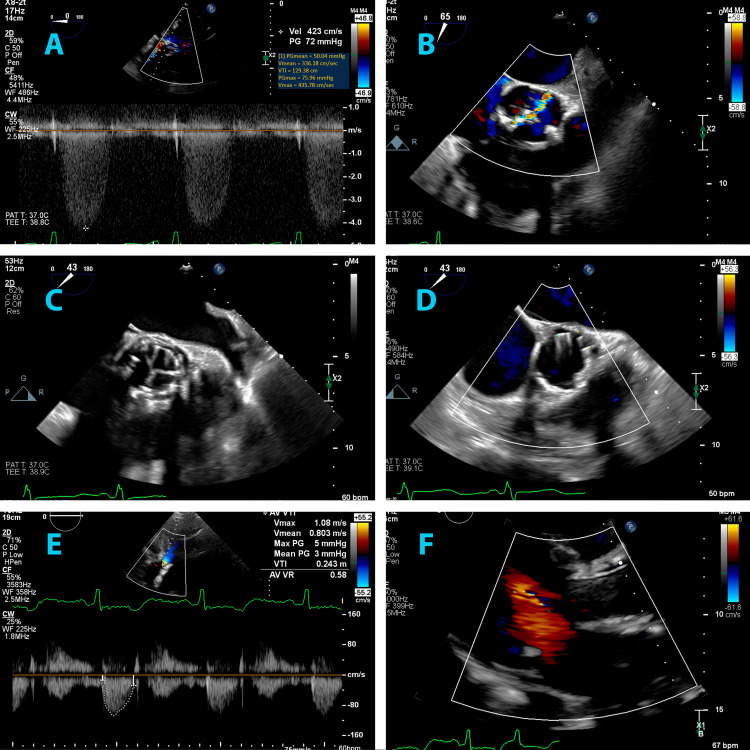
Intraprocedural TEE and post-procedural TTE A - Intraprocedural TEE confirming velocities consistent with severe aortic stenosis, B - intraprocedural TEE showing aortic regurgitation, C - intraprocedural TEE showing TAVR post-deployment, D - intraprocedural TEE showing lack of valvular or paravalvular regurgitation, E - post-procedural TTE showing normalized aortic valve velocities and gradients, F - post-procedural TTE showing lack of valvular or paravalvular regurgitation TEE: transesophageal echocardiogram; TTE: transthoracic echocardiogram; TAVR: transcatheter aortic valve replacement
